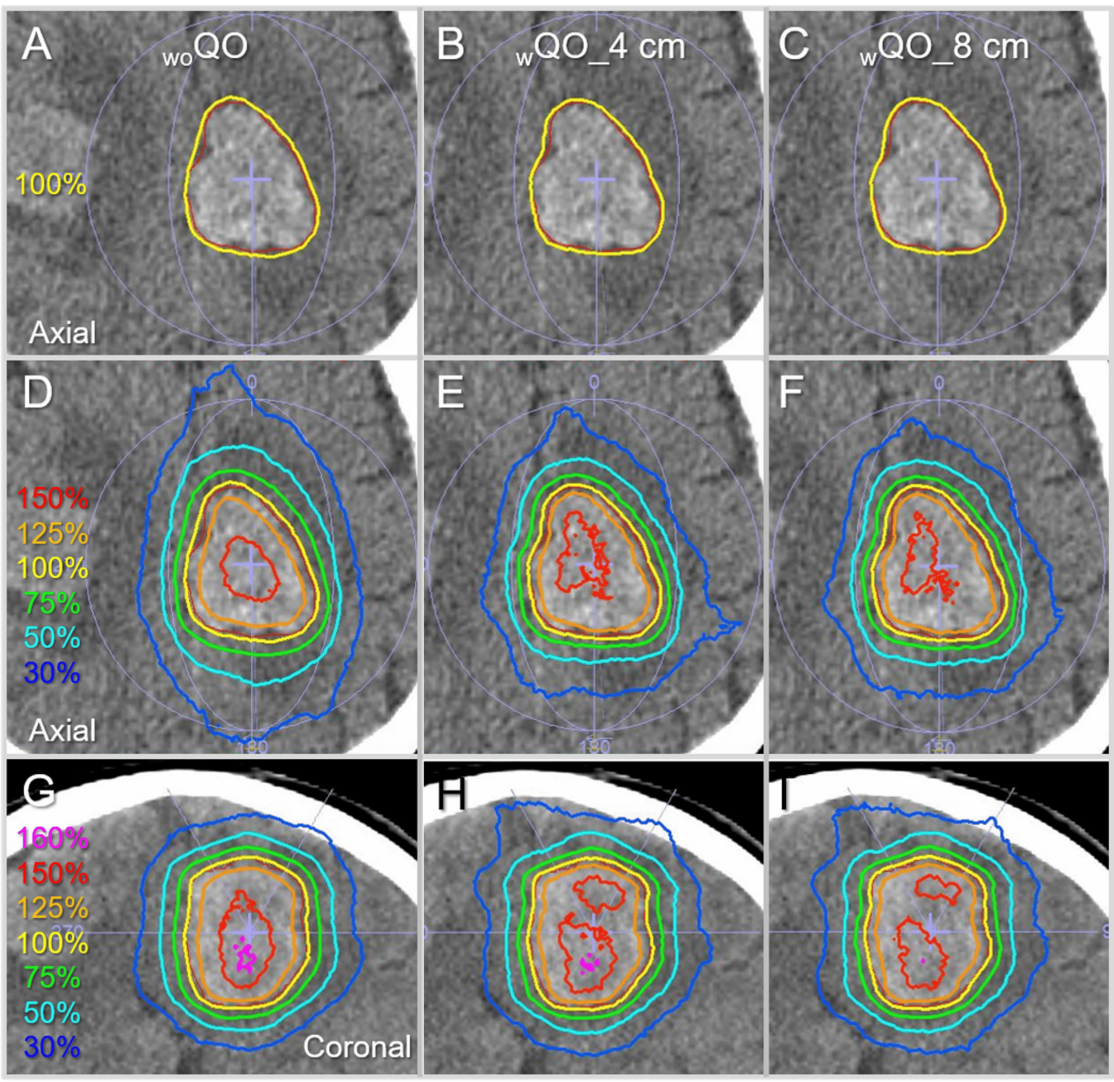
Comparison of dose distributions for single brain metastasis with a GTV of 28.88 cc. The images show head computed tomography images of a patient harboring multiple brain metastases including the lesion in the left fronto-parietal lobe with the GTV of 28.88 cc (A-I), onto which the GTV contoured in red, arc arrangements, and representative isodoses are superimposed; axial views  (A-F); and coronal views (G-I). The isodose lines are shown as relative values with the GTV *D*_V-0.01 cc_ (*D*_99.97%_) as 100% (yellow). _wo_QO: without Quadratic Overdose; _w_QO_4 cm; with Quadratic Overdose with 4 cm margin around target (MAT); _w_QO_8 cm; with Quadratic Overdose with 8 cm MAT; GTV: gross tumor volume; *D*_V-0.01 cc_: a minimum dose covering a target volume minus 0.01 cc.
